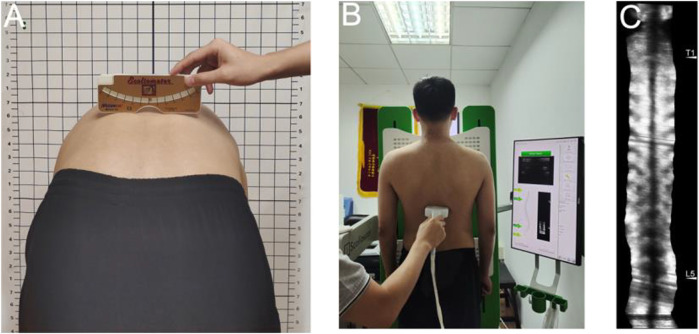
Scoliosis screening methods-(A) Adam’s test and trunk rotation angle measured with a Scoliometer; (B) A radiation-free spine ultrasound imaging system; (C) Ultrasound imaging.
